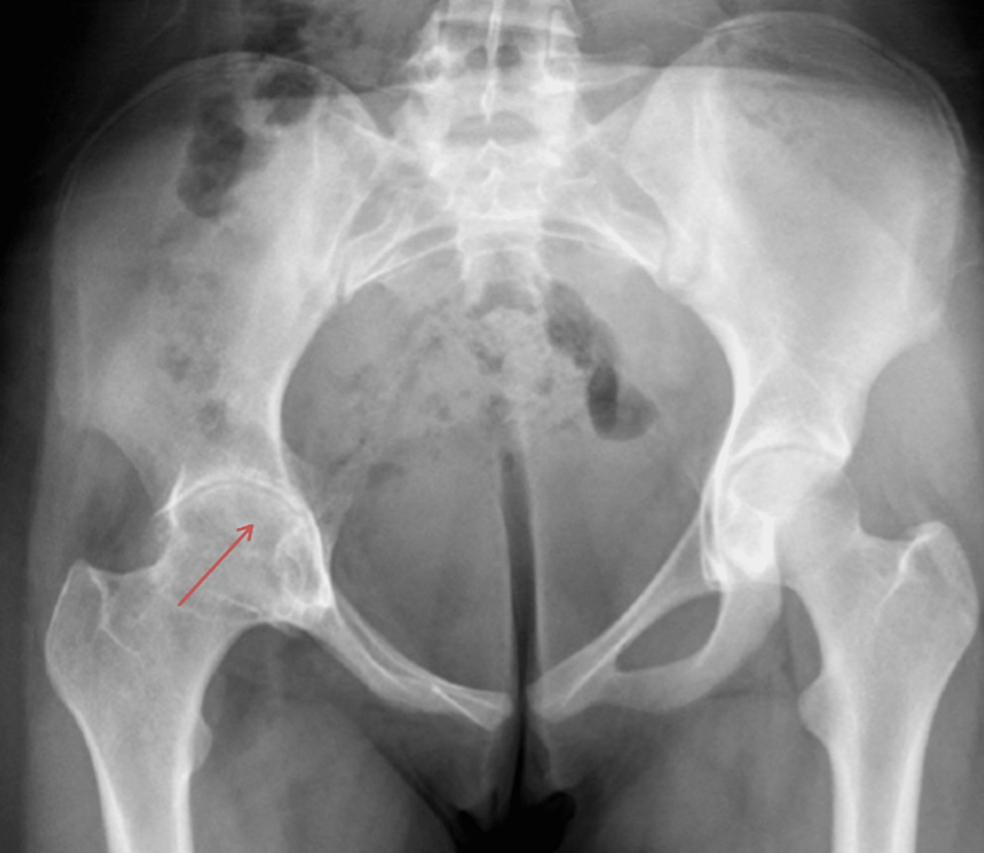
Follow-up pelvis anteroposterior radiograph showing concentric joint space narrowing on the right side with increased central edge angle with a mild pelvic tilt to the right side (red arrow).
